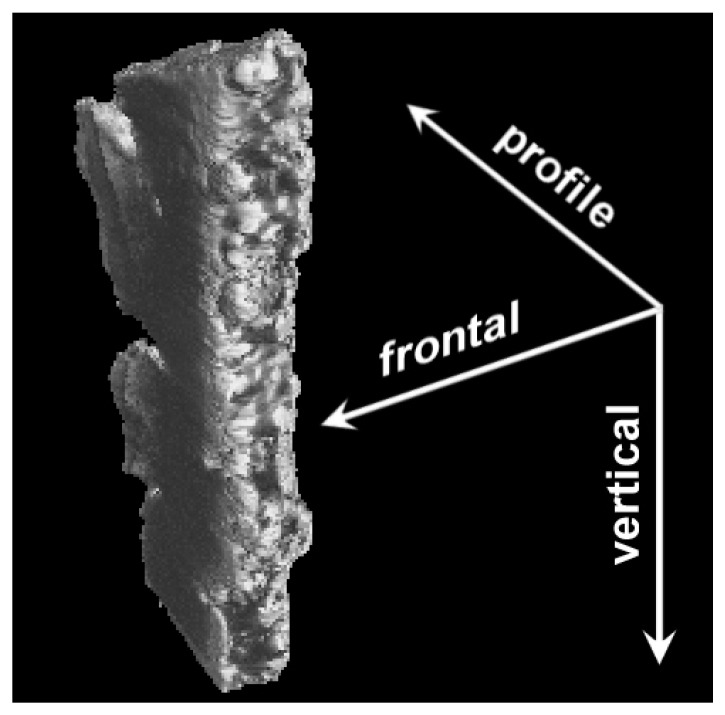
The sample’s scanning directions by X-ray tomography.
